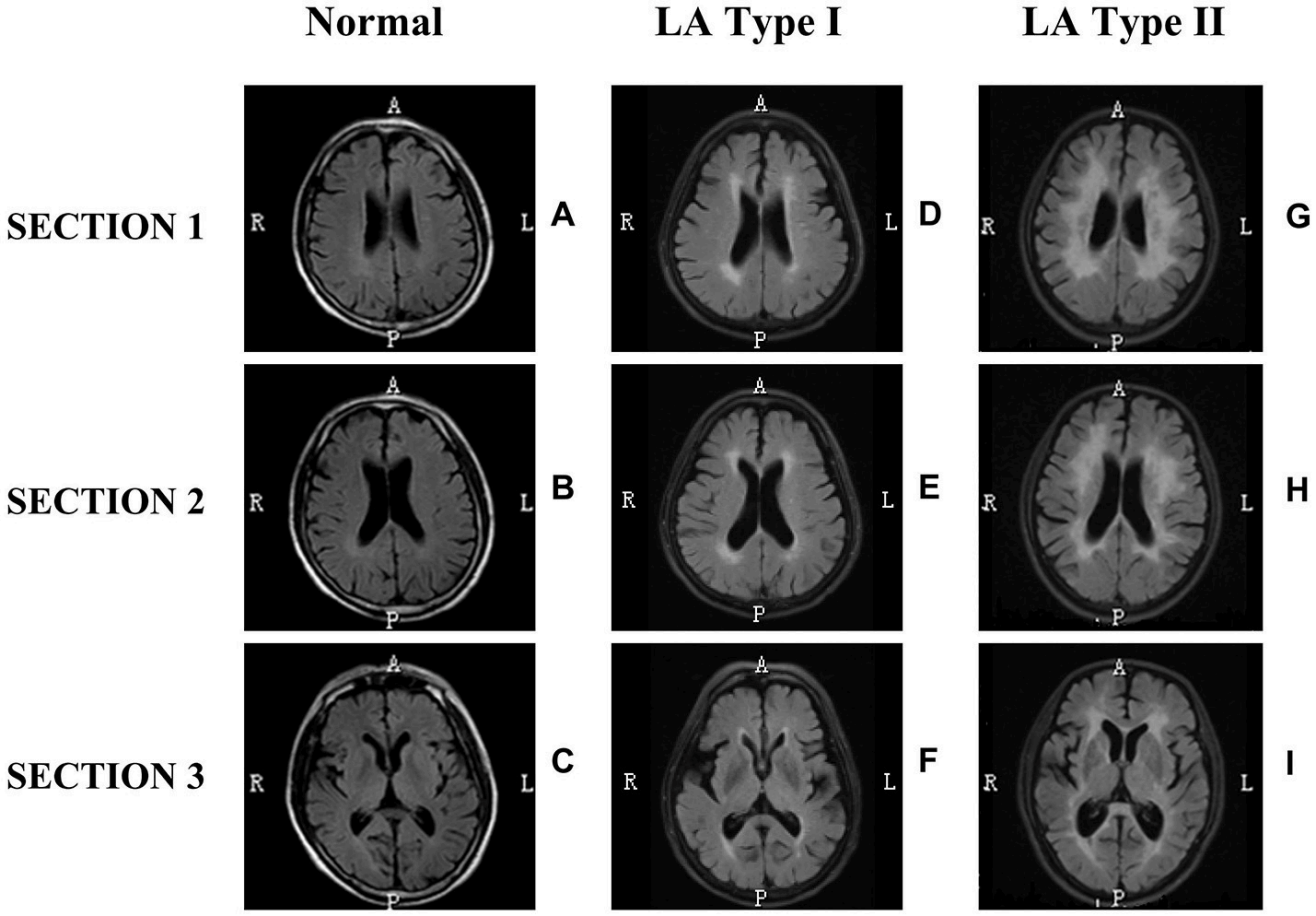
Neuroimaging classification of leukoaraiosis (LA). LA was defined according to the systematical assessment of white matter lesions on three section (1, 2, 3) of neuroimaging of subjects (D,E,F or G,H,I). LA type I (D,E,F) and LA type II (G,H,I) showed white matter hyperintensity on all the three sections of T_2_-weighted FLAIR MRI scans, but not on Normal (A–C). Sections 1, 2, 3 represent the centrum semiovale section, lateral ventricles section, and internal capsule section, respectively. The first column shows few white matter lesions on all three sections of MRI imaging. The second column shows white matter changes including a pencil-thin lining or smooth halo along the side of the lateral ventricles, small periventricular caps around the frontal horns of the lateral ventricles, with early confluent lesions. The third column shows irregular periventricular lesions, largely extending periventricular caps, and severely confluent abnormalities. In addition, this classification scheme was proposed to divide LA-associated neuroimaging phenomenon into three broad categories: Normal (A–C), LA type I (D–F) and LA type II (G–I), based on both the severity of white matter lesions and new definition of LA. This classification method of LA neglected the neuroimaging distinctions between periventricular WMLs (PVWMLs) and deep/subcortical WMLs (DWMLs) and reduced the heterogeneity for helping clinicians and neuroscientists easily to diagnose LA and to evaluate severity precisely. It's helpful for scientists to decipher the pathogenic mechanism and clinically functional consequence properly.
